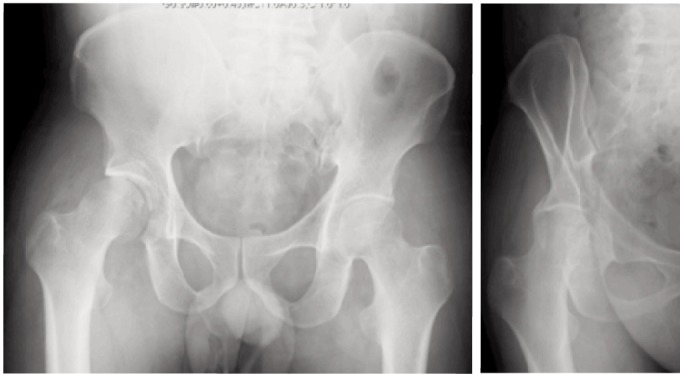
Initial radiographs of Case Two showing a Transverse-posterior wall fracture of the right acetabulum.
